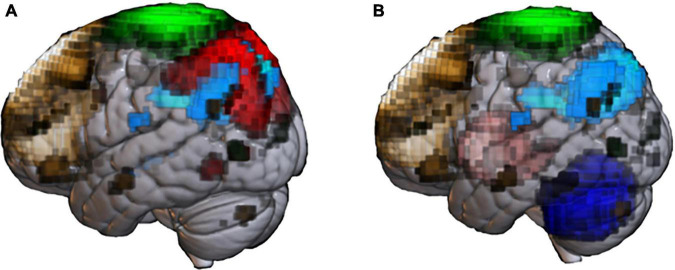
Abnormal resting-state networks in Alzheimer’s disease (AD) and Parkinson’s disease (PD). Functional network templates were extracted from the MRI database (70 healthy elderly) at the National Center of Neurology and Psychiatry’ to display network abnormality reported in past AD (A) and PD (B) studies. (A) Default mode network (DMN, light blue) is the representative network impaired in patients with senile-onset AD. Early- onset AD shows impaired not only DMN, but also executive control network (brown), sensory-motor network (SMN, green) and dorsal-attention network (red). (B) Patients with PD shows extensive network impairment, including basal ganglia (pink), cerebellum network (blue), and SMN. PD patients with cognitive impairment shows impaired DMN and ECN.
